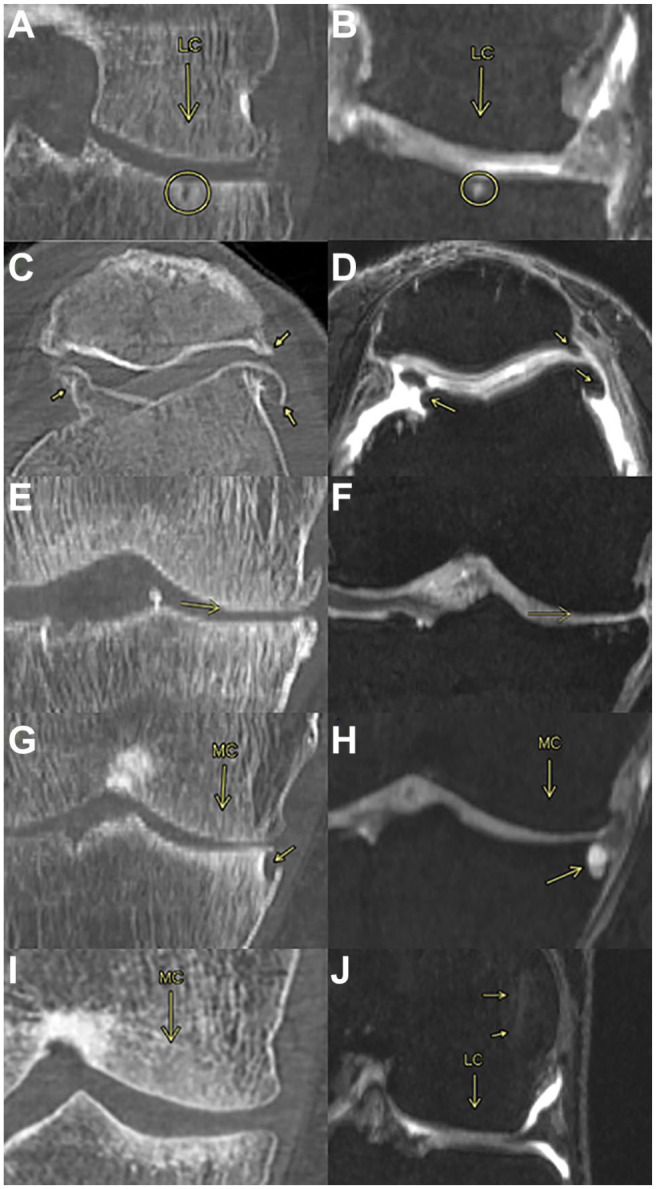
Bone observations. (A, B) Subchondral bone cysts are typically spherical or ellipsoidal fluid-filled cavities within the subchondral bone region. (C, D) Osteophytes are cartilage-capped bony proliferations (spurs) that most commonly develop at the margins of a synovial joint as a response to articular cartilage damage. (E, F) Bone attrition is the result of flattening or depression of the articular surfaces, probably because of bone remodeling. (G, H) Osteonecrosis is a generic term referring to the ischemic death of the constituents of the bone and is observed as if the bone is missing a piece. (I) Subchondral bone sclerosis is a thickening of the bone seen in joints affected by OA. It is observed as a “whitening” of the bone only in CT. (J) Subchondral bone edema is a build-up of fluid in the bone marrow as a response to an injury or OA condition visible on MRI but not on CT. MRI = magnetic resonance imaging; OA = osteoarthritis; CT = computed tomography.
